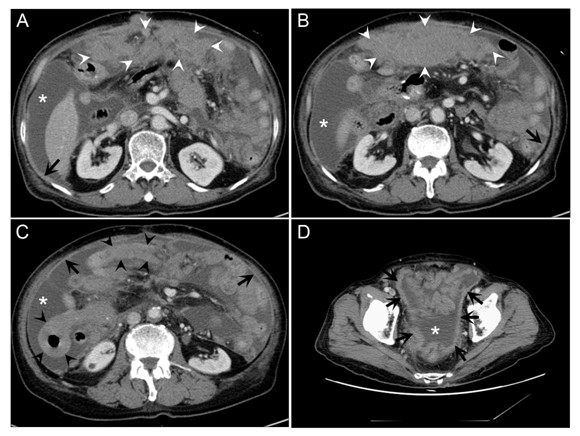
81-year-old man with a peritoneal lymphomatosis by diffuse large B-cell lymphoma. CT scan images (A-D) show diffuse peritoneal thickening (black arrows), diffuse nodular infiltration of omentum (white arrowheads), bowel wall thickening (black arrowhead) and ascites (asterisk).
